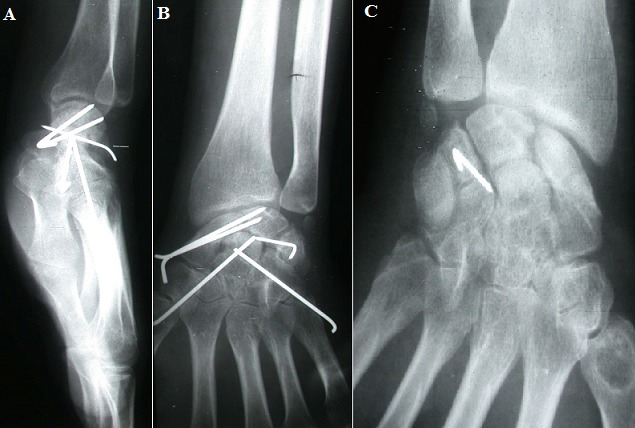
(A,B, C) radiographies du poignet (face, profil): consolidation osseuse des os du carpe
